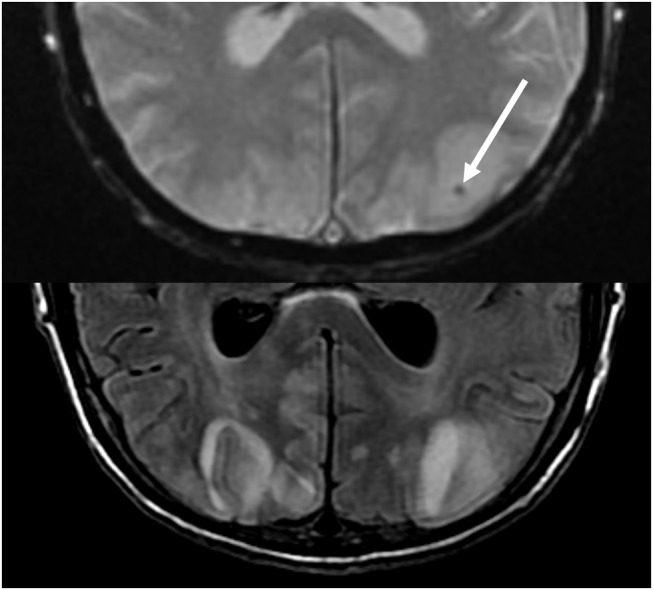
Patient with a prior liver transplantation on Tacrolimus. T2 gradient recalled echo (top) demonstrates a focal area of hemorrhage within the vasogenic edema in the left occipital lobe. T2-FLAIR demonstrates the more typical finding related to PRES with signal abnormality in the bilateral occipital lobes.
